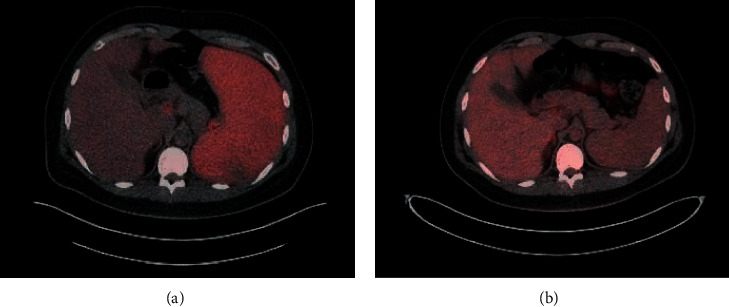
PET/CT comparison after 4 weekly dose of rituximab. Pretreatment PET/CT (a) showing FDG-avid splenomegaly. PET/CT after 4 weekly doses of rituximab 375 mg/m^2^ (b), demonstrating a complete response.
